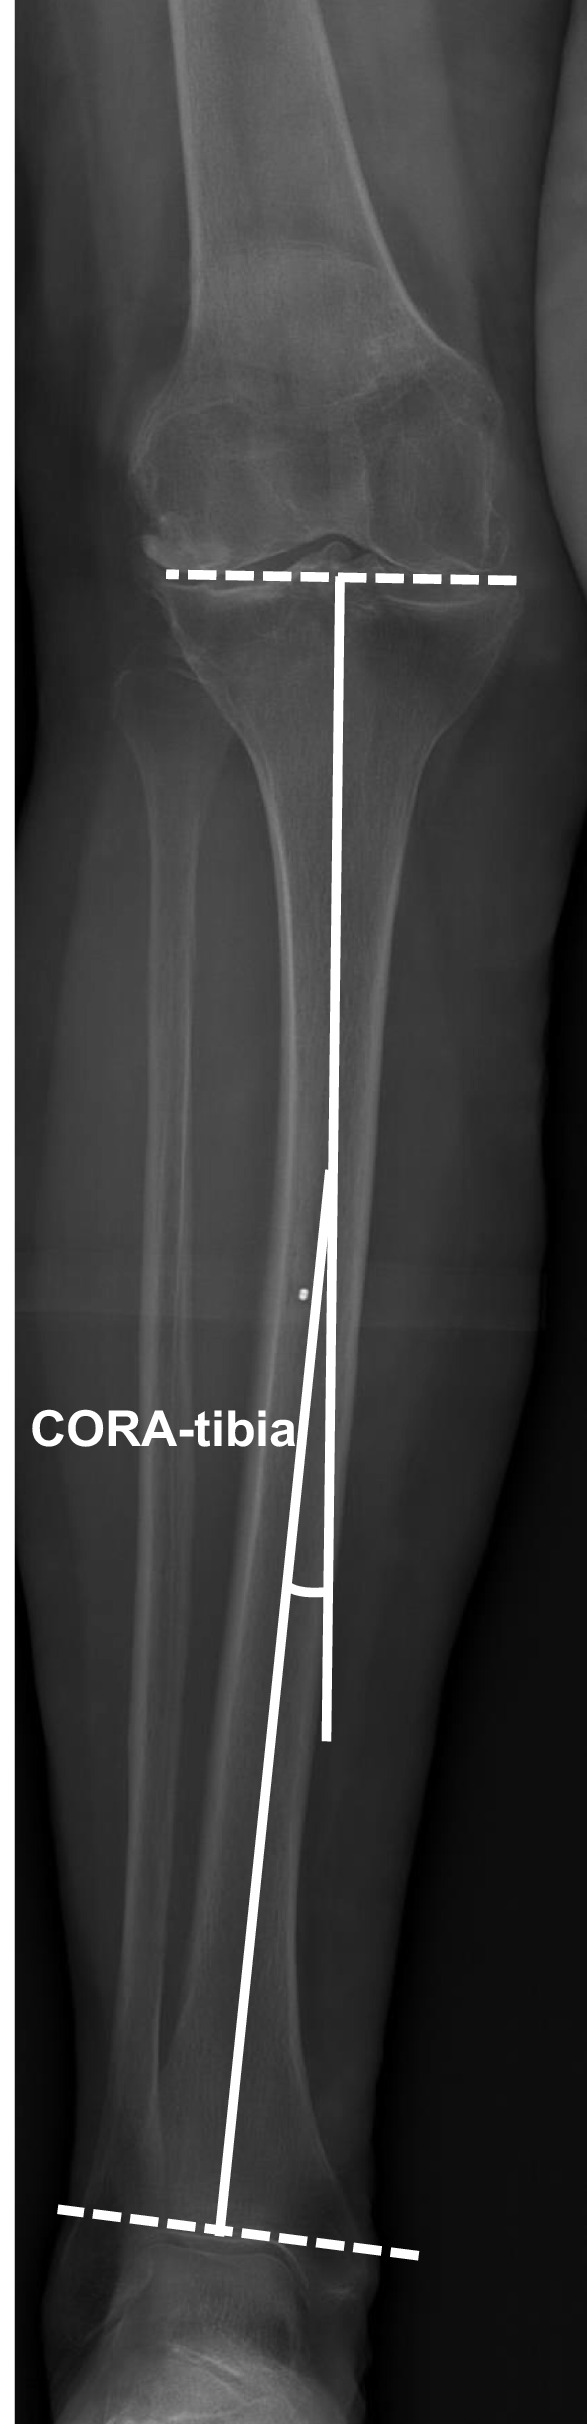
EOS radiograph demonstrating measurement of extra-articular tibial deformity in valgus knee. Close-up of the knee joint and leg of a pre-operative, weightbearing lower-limb anteroposterior EOS digital radiograph is shown. The angle formed by the proximal and distal tibial mechanical axes is the centre of rotation angulation (CORA-tibia)
